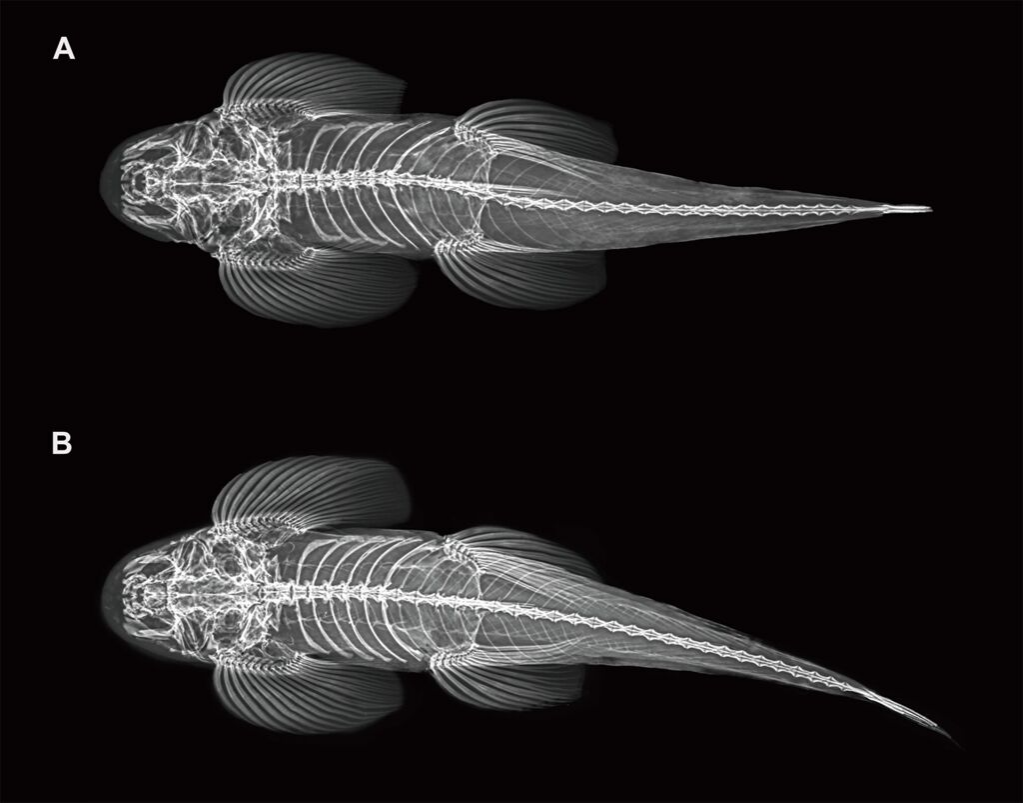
Dorsal profile of the skeleton of of *Balitorayingjiangensis* shown under X-ray; A KIZ1995000888 (Holotype); B KIZ1995000889 (Paratype).
